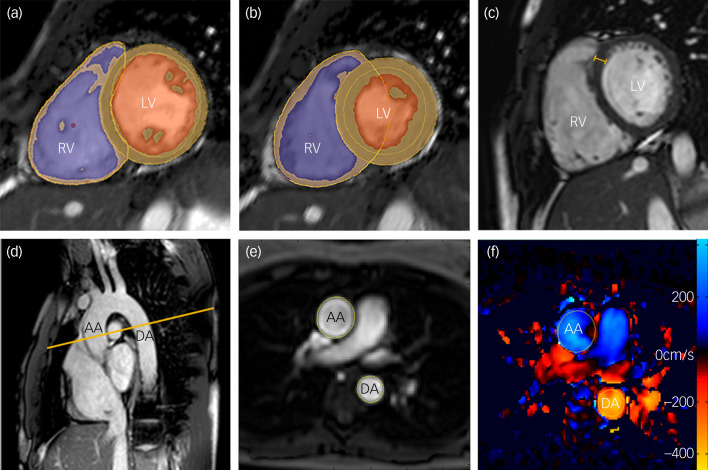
Magnetic resonance images demonstrating the assessment of biventricular volumes and function in an adult participant.
